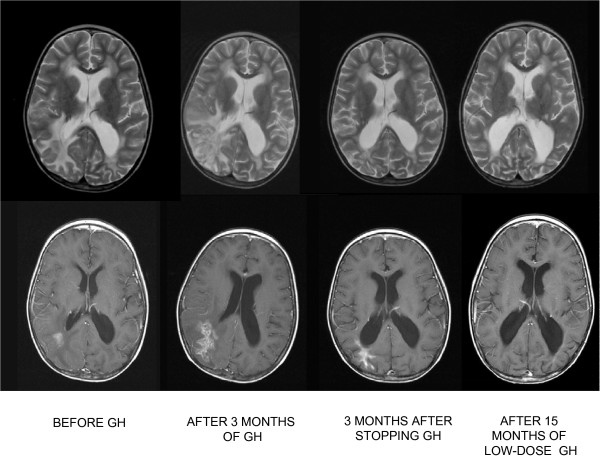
Iconography strongly suggests an association between growth hormone administration and proinflammatory activity. Axial MRI images immediately before administering growth hormone, after 3 months of growth hormone administration, 3 months after suspending growth hormone, and after 15 months of lower-dose administration of growth hormone. The areas of focal enhancement in the images taken before the therapy with growth hormone reveal inflammation and necrosis, an expression of post-therapy alterations. After 3 months of growth hormone therapy, the main finding is an increase in the perifocal edema occupying the posterior half of the right hemisphere, which decreased after suspending growth hormone. After 15 months of low-dose administration of growth hormone, there is no evidence of further edema in the post-therapy radiological alterations.
